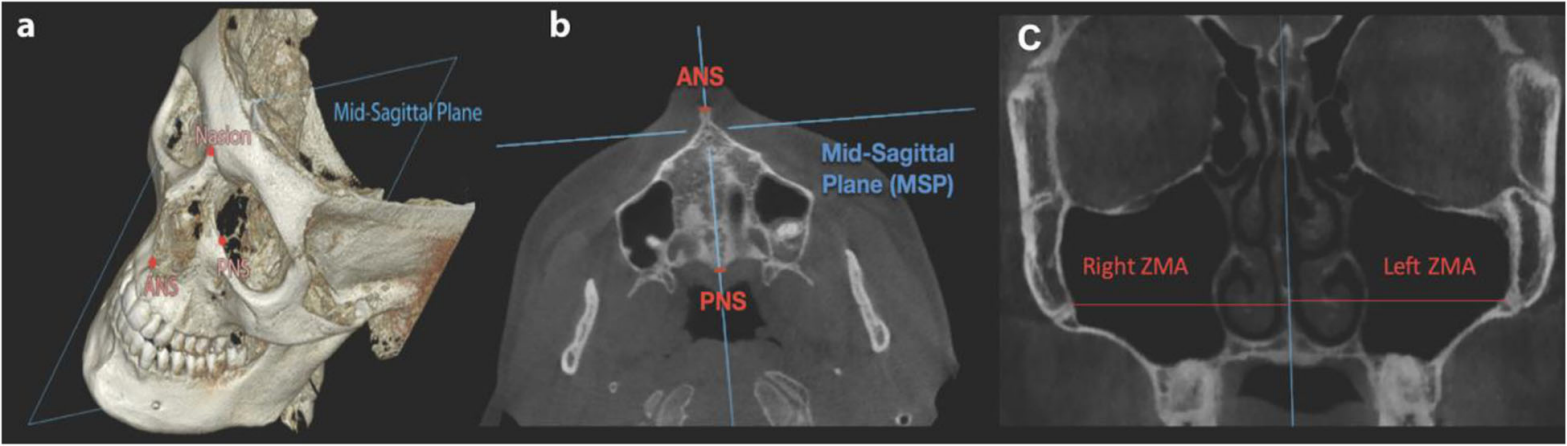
a CBCT image showing mid-sagittal plane (MSP) on subject’s initial CBCT using OnDemand (Cybermed, Korea). ANS, PNS, and nasion can be viewed as separate skeletal landmarks on the MSP. b Axial slice at pre-expansion with vertical line passing through ANS and PNS. c Coronal view of pre-expansion CBCT displaying measurements from the MSP to both the right and left ZMA. Right and left ZMA landmarks indicated in red at the most medial-superior location of the zygomatic-maxillary suture
